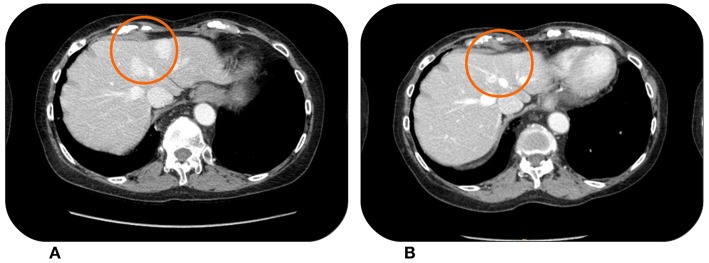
This patient showed hypervascular liver metastases from retroperitoneal leiomyosarcoma at the baseline CT scan in April 2015 (A). The lesions were reduced in both size and vascularisation after treatment with Trabectedin, which was stopped in December 2016 (B) for a drug holiday.
